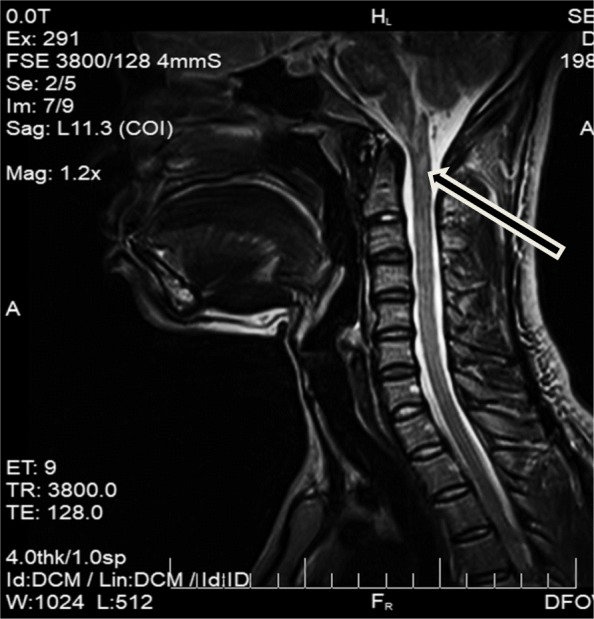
Cervical medullary magnetic resonance imaging showing an additional intramedullary hypersignal lesion at C2 level (arrow) in T2 sequence 3 months later
